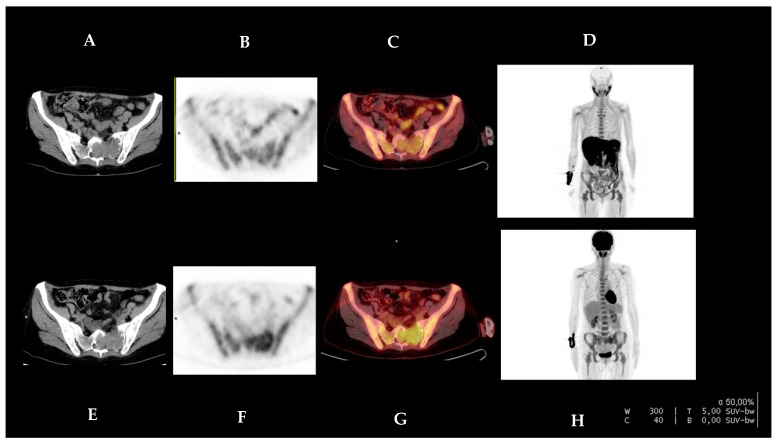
Sixty-seven year-old female (patient #3) presented with plasmacytoma in left sacrum. [^18^F]FCH PET/CT (upper row, (A–D)) and [^18^F]FDG PET/CT (lower row, (E–H)) are presented, corresponding to the CT, PET, PET/CT fusion, and MIP (maximum intensity projection). The images show higher uptake with [^18^F]FDG than with [^18^F]FCH (SUVmax 4.5 vs. 4.1, respectively) in the lesion in the left sacrum. The [^18^F]FCH PET/CT and [^18^F]FDG PET/CT images are presented with equal grey-scale intensity.
